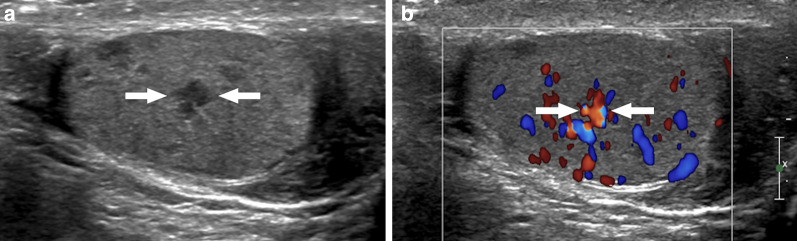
Leydig’s cell hyperplasia. A 34-year-old male presenting with infertility underwent testicular US exam for primary evaluation. a Gray-scale US image shows several hypoechoic lesions scattered throughout the parenchyma in both testes. The largest lesion was in the right testis (arrows). Both testes were deemed to be small in size when patient’s age was considered. b Color Doppler US image demonstrates intense vascularity within the largest lesion. Provisional diagnosis of Leydig’s cell hyperplasia was made. Genetic analysis confirmed Klinefelter’s syndrome
